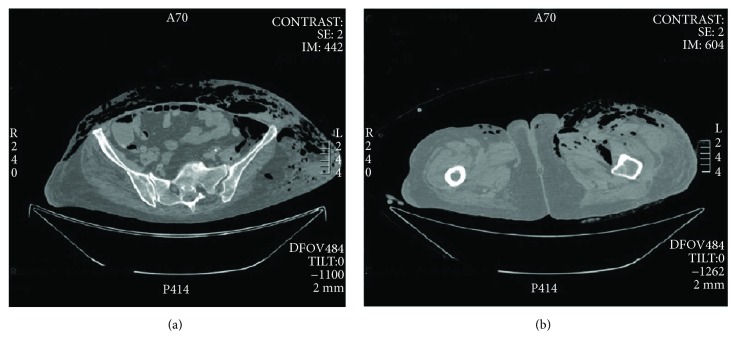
(a) Abdominal CT scan detecting intraperitoneal free air. (b) Subcutaneous emphysema of the left thigh root.
